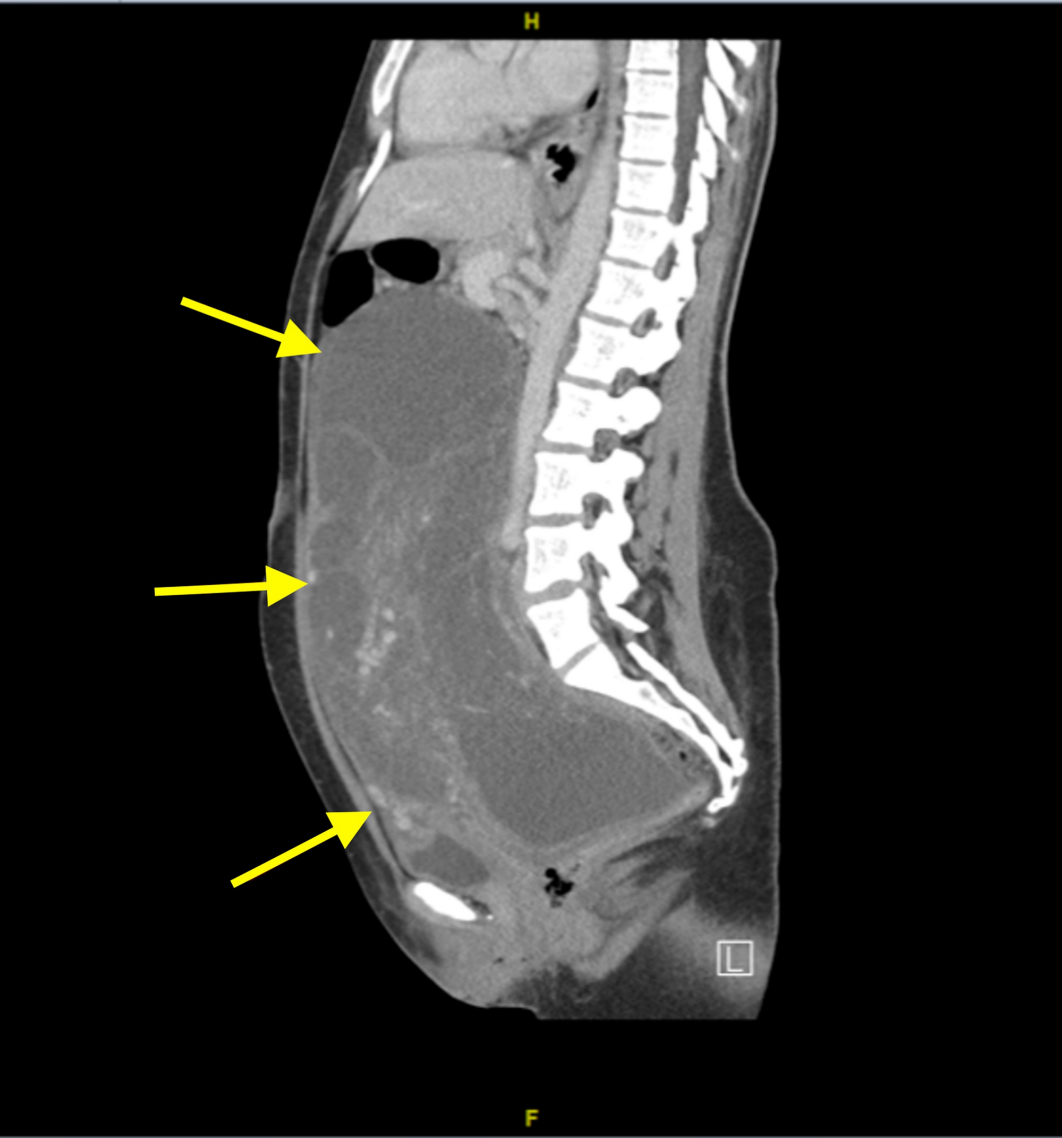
CT sagittal view of the abdomen and pelvis Showing the large mass extending from the pelvis to the abdomen.
